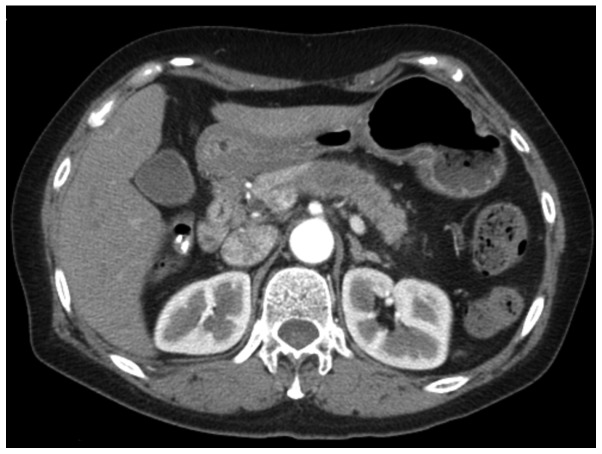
Pre-operative CT image of the pancreas. Enhanced CT showing a hypovascular tumor, 2 cm in diameter, in the pancreatic body. CT, computed tomography.
